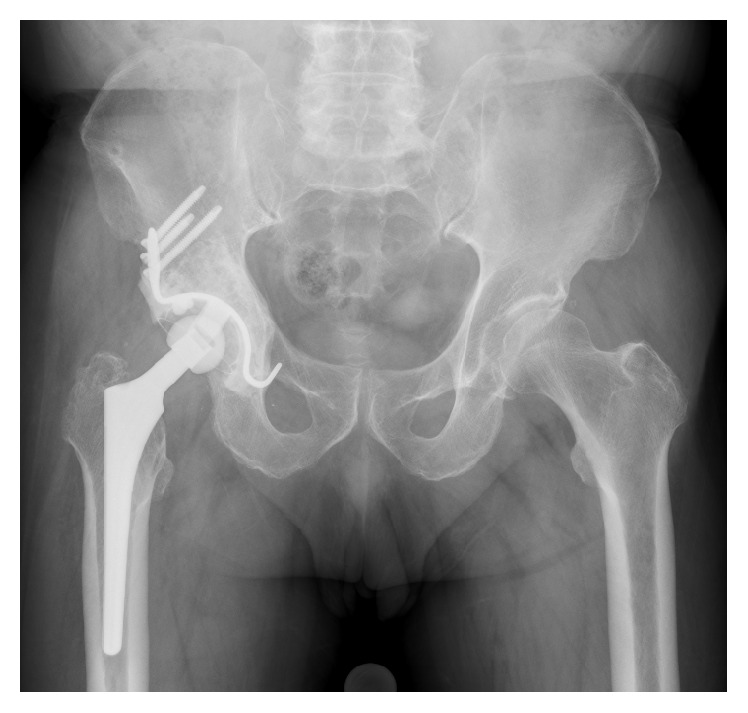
An anteroposterior plain radiograph, obtained 4 years after the rerevision surgery, showing acetabular reconstruction performed with a Kerboull-type acetabular reinforcement device and bulk structural allograft.
